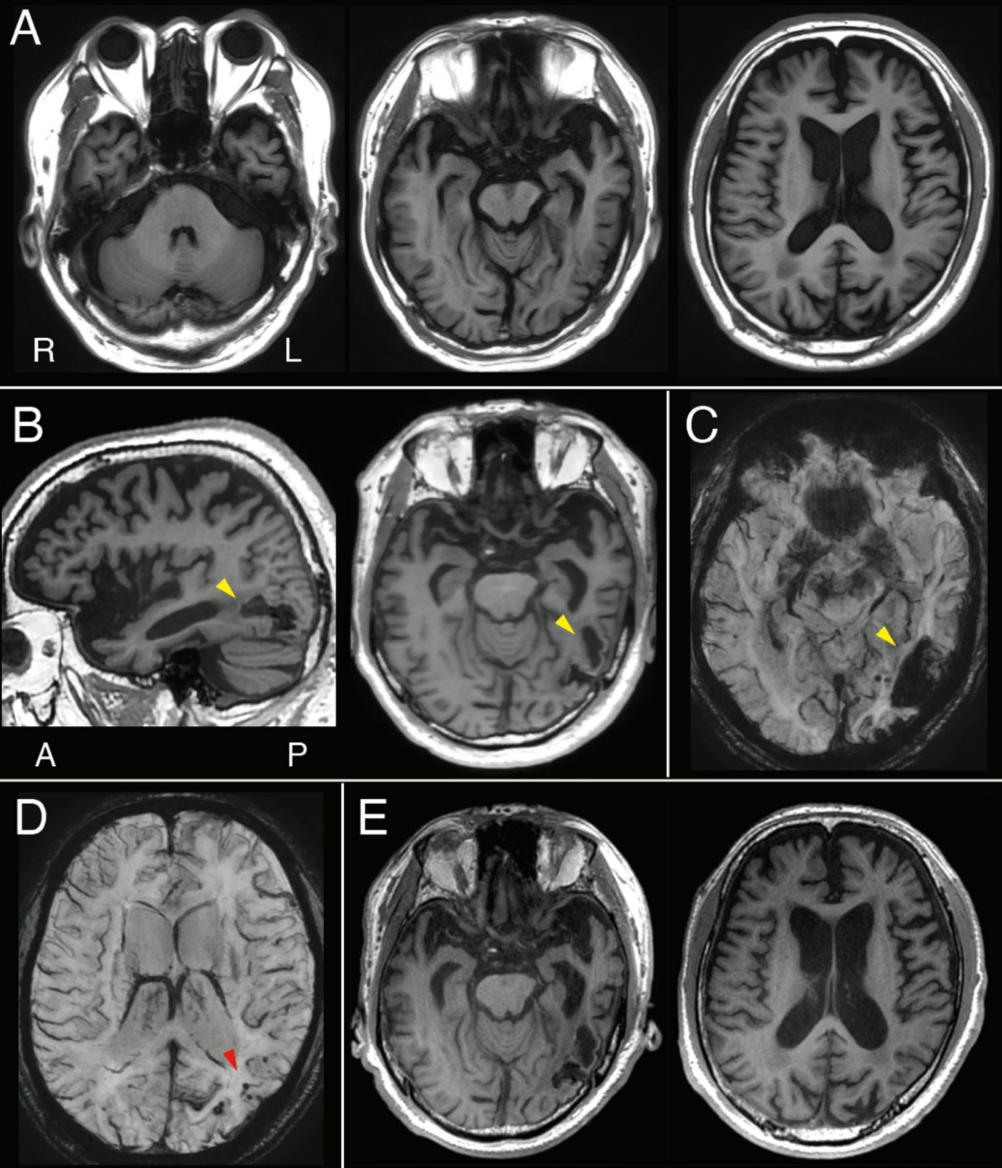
Neuroradiological findings. A: Magnetic resonance imaging (MRI) conducted eight months before revealed mild diffuse atrophy without evidence of cerebral hemorrhage. B: Follow-up MRI shortly following the initial visit showed subcortical hemorrhage in the left posterior inferior temporal region, which was absent on the earlier MRI (yellow arrow). C, D: Susceptibility-weighted imaging (SWI) confirmed the hemorrhage in the same region (yellow arrow) and additionally revealed multiple microbleeds, predominantly in the left parietal, occipital, and temporal cortices (red arrow). E: Follow-up MRI conducted 20 months following the initial visit showed progressive atrophy in the bilateral medial temporal lobes
